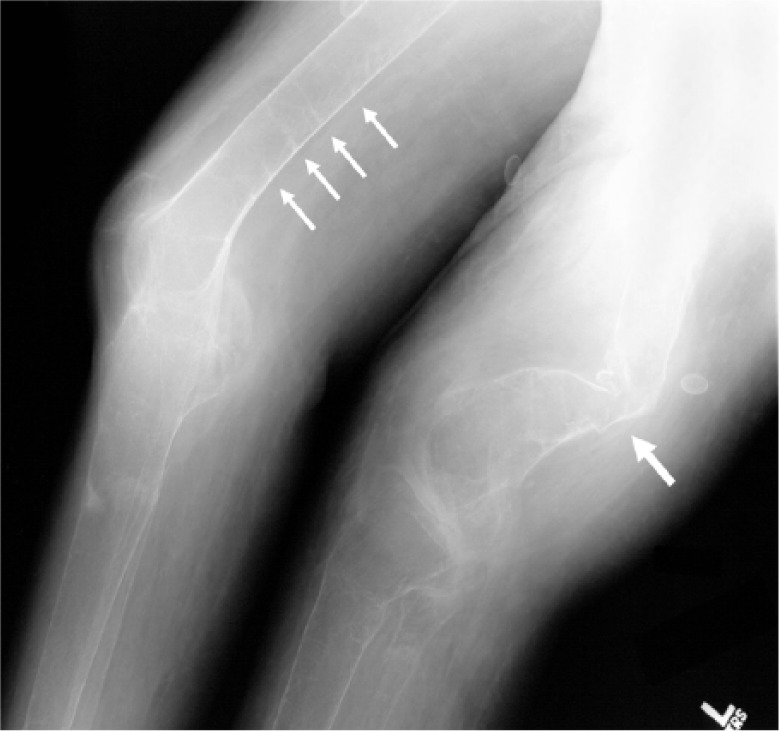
A radiograph of the distal femurs shows further evidence of badly malformed bones secondary to severe osteomalacia (large arrow), as well as several additional pseodofractures (small arrows).
